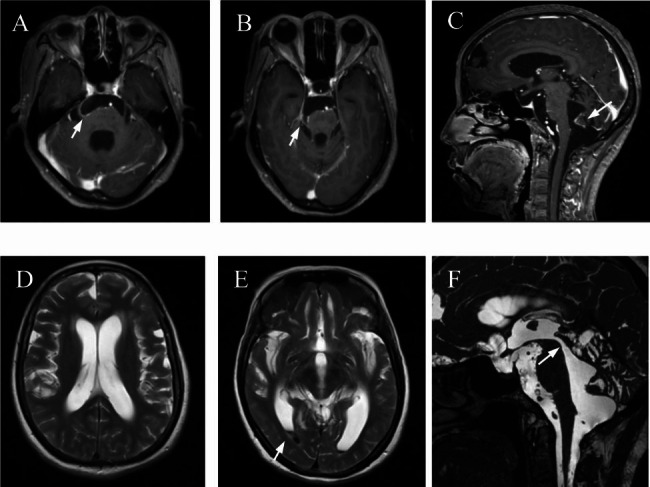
Manifestations of meningitis and hydrocephalus in the patient. A: T1-weighted image with contrast enhancement reveals enhanced pia mater in the right cerebellar pontine angle cistern. B: T1-weighted image with contrast enhancement shows enhanced pia mater in the right pontine cistern. C: T1-weighted image with contrast enhancement in sagittal position reveals enhanced pia mater in the fourth ventricle. D: T2-weighted image shows enlarged lateral ventricle. E: T2-weighted image shows hemorrhage in the occipital horn of the right lateral ventricle. F: T2-weighted image in sagittal position shows unobstructed cerebral aqueduct of midbrain, suggesting communicating hydrocephalus
